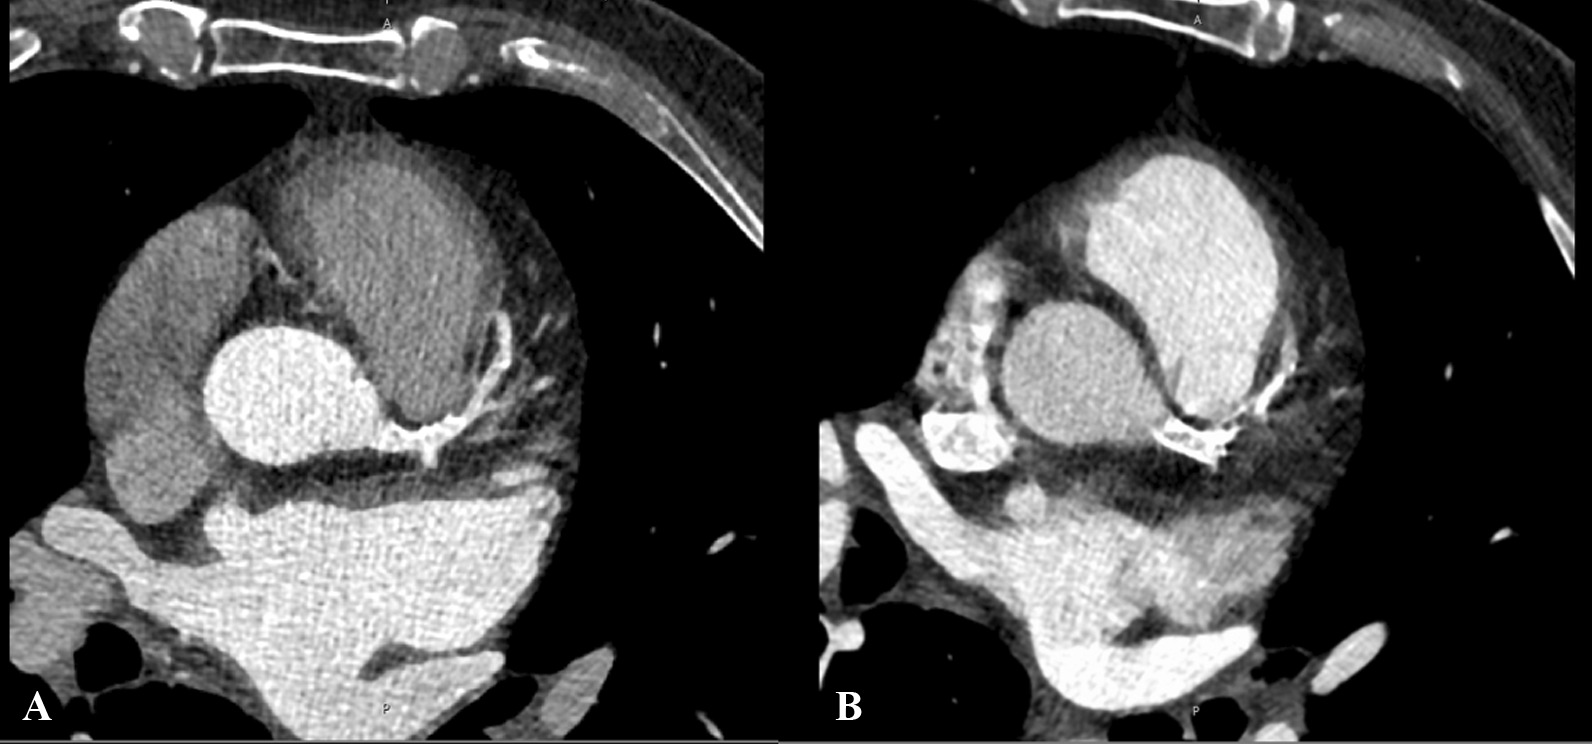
CT-Scan in 2017 A and 2021 B (CT-Siemens—SOMATOM Force)
